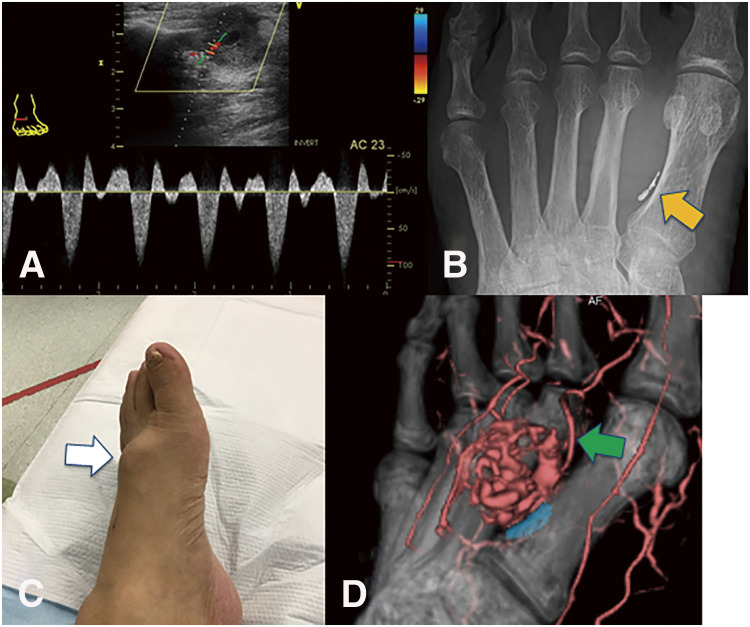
Post-embolization findings. (A) Echography performed 3 months after embolization reveals recurrence of the first dorsal metatarsal artery aneurysm with to-and-fro blood flow waveform. (B) The radiograph indicates no coil migration (orange arrow). (C) Nine months after embolization, the mass on the dorsal foot returned (white arrow), with a recurrence of pain. (D) Contrast-enhanced computed tomography reveals that the distal first dorsal metatarsal artery (green arrow) supplies the aneurysm.
